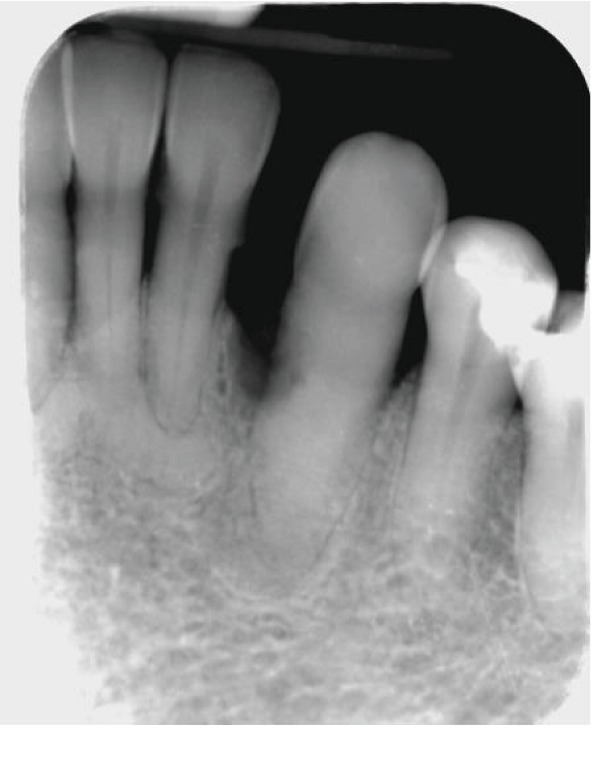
Long-cone periapical radiograph of the LL3 showing loss of about 50% bony support, obliteration of the root canal, radiolucency on the mesial surface of the root, and sclerosing osteitis periapically. No signs of caries intraorally. The LL2, LL1, and LR1 show 50-70% bone loss and bony sclerosis apically which could indicate late-stage periapical cemento-osseous dysplasia.
